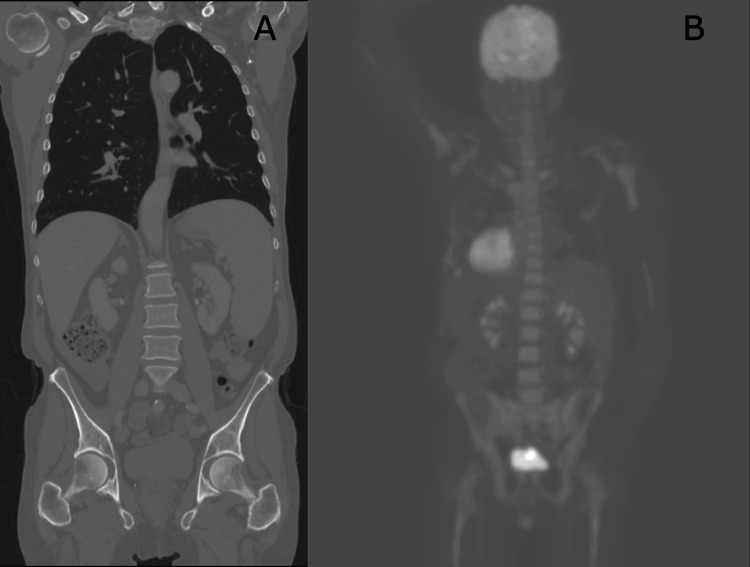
A) CT of chest, abdomen, and pelvis demonstrating splenomegaly and borderline hepatomegaly. B) Positron emission tomography demonstrating hypermetabolism in the axial and proximal appendicular skeleton.
